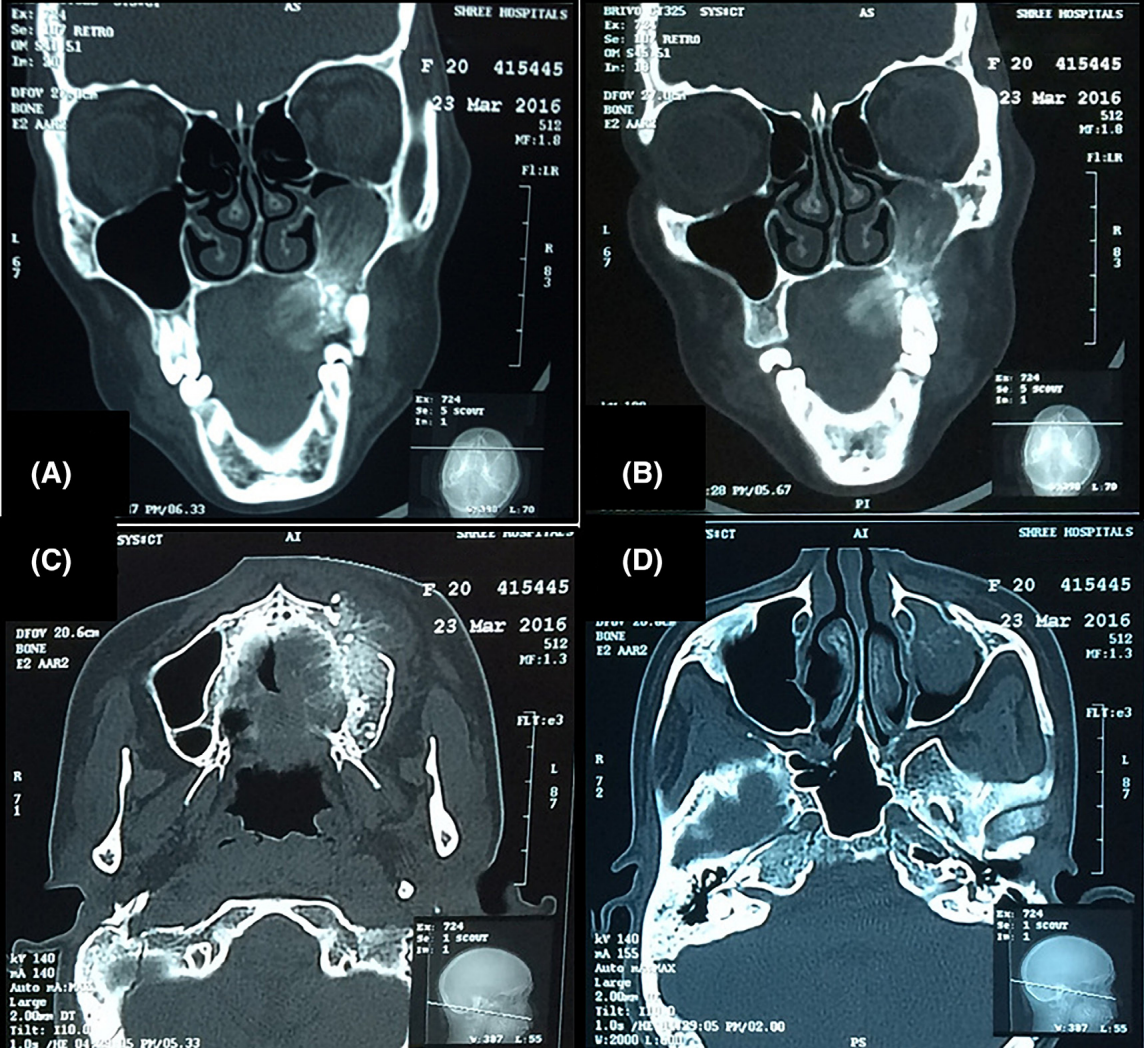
(A and B) Coronal CT scan showing hyperdense mid‐facial mass involving the left maxillary sinus. (C and D) Axial CT scan view showing mixed‐density mass with expansion of the left palate with diffuse borders.
